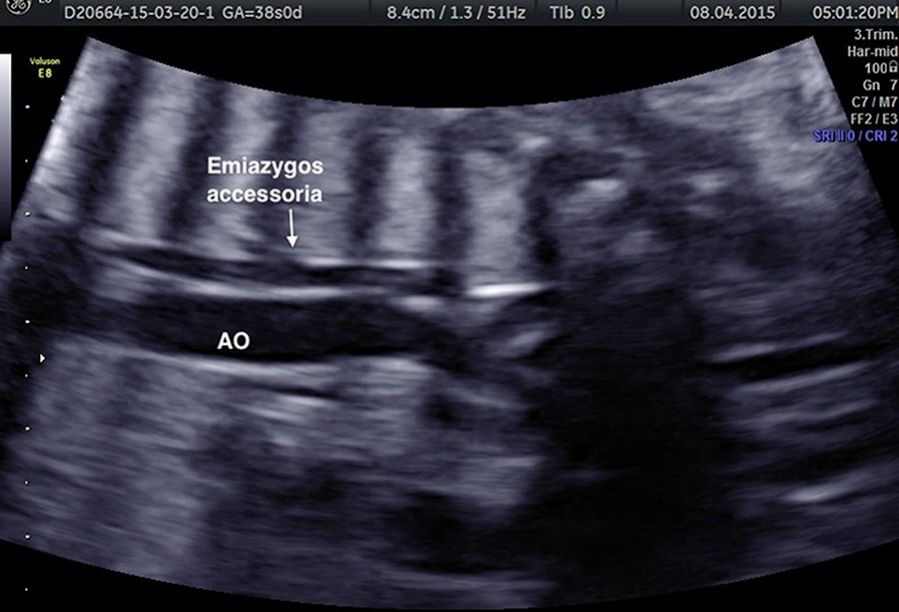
The prenatal ultrasound shows a vessel with parallel course on the left and posteriorly to the thoracic aorta
